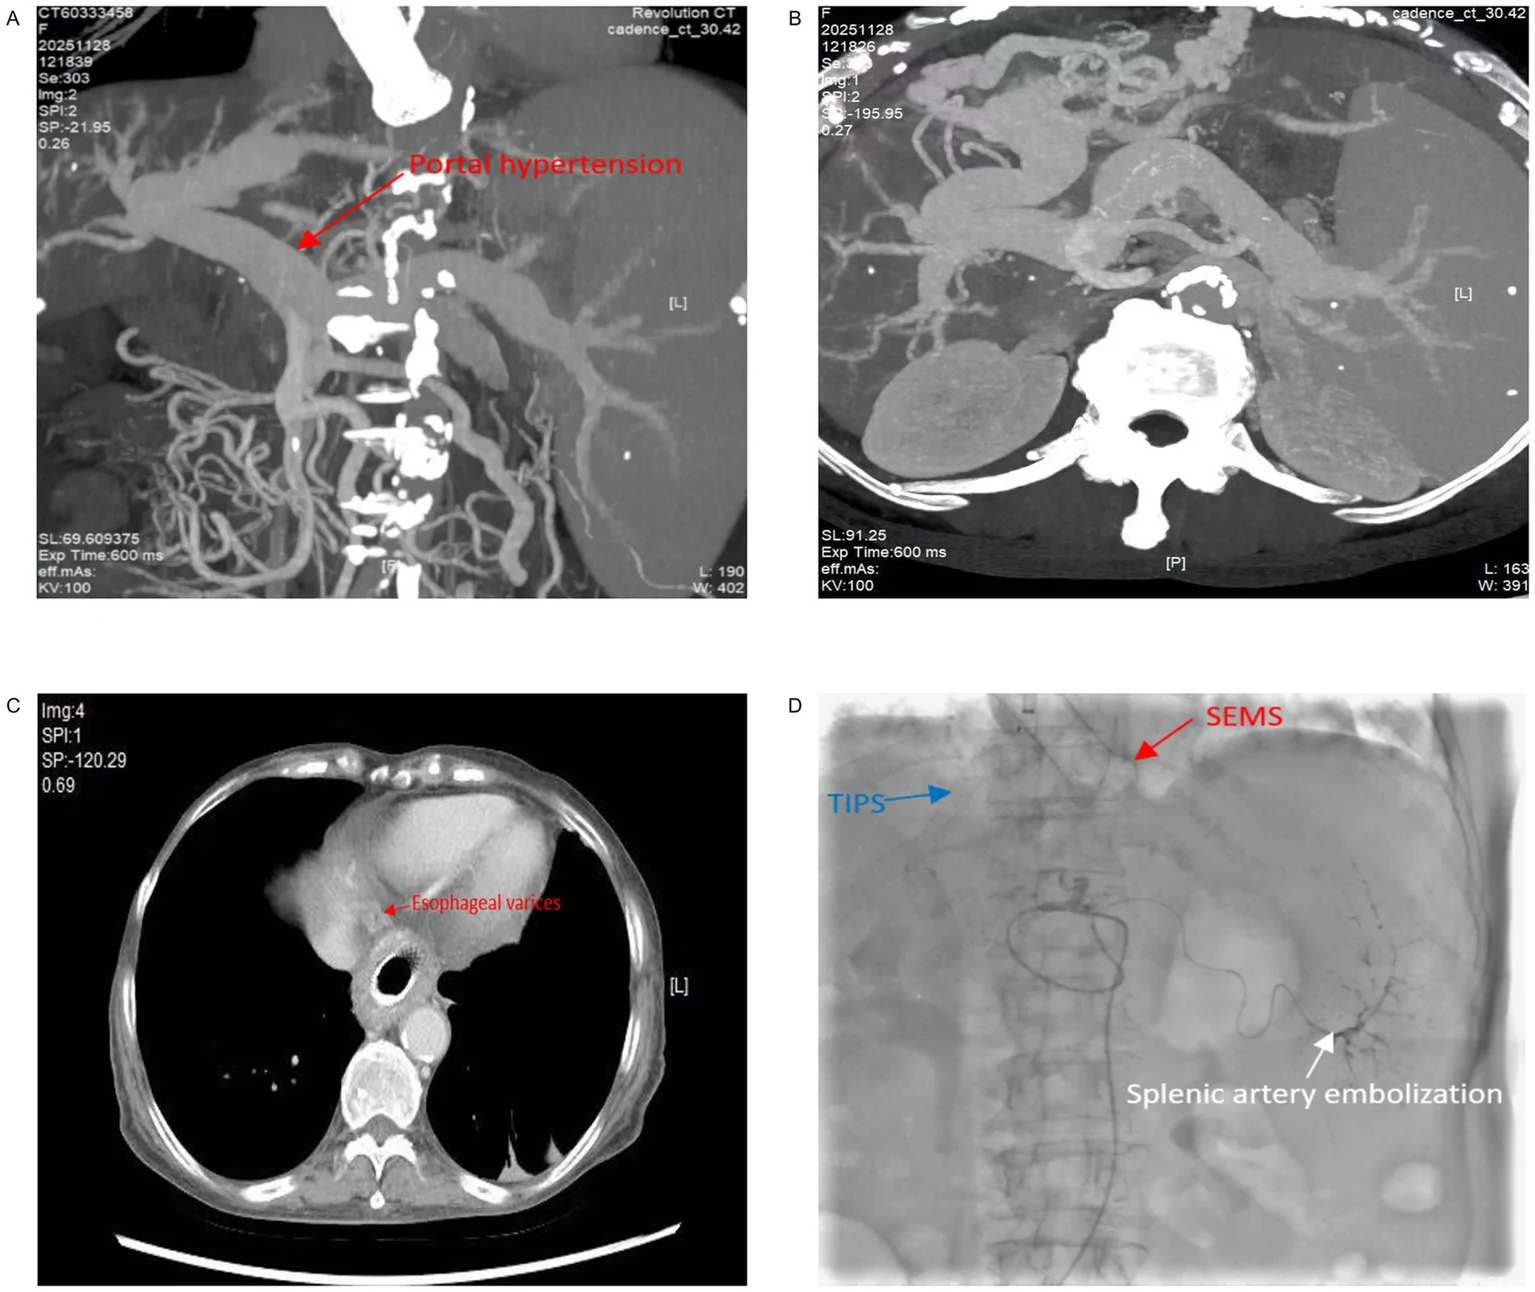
Contrast-enhanced CT scans findings before the TIPS procedure. (A) Pre-TIPS portal vein CT venography (CTV) review; (B) The portal vein and its branches; (C) Esophageal varices; (D) Post-TIPS imaging.
